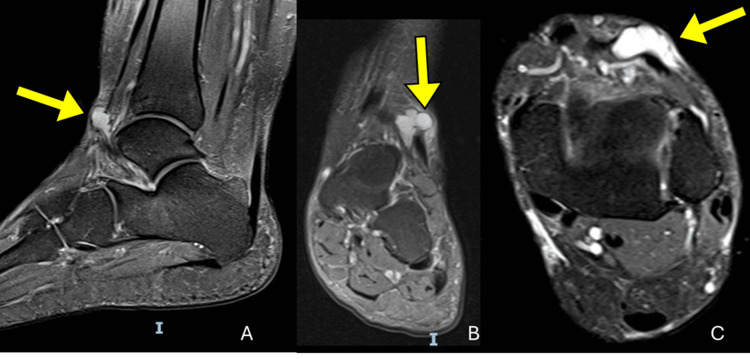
Proton density fat-saturated (PD-FS) MRI of the left ankle demonstrating a ganglion cyst (yellow arrows). (A) Sagittal, (B) coronal, and (C) axial PD-FS images show a well-defined, multilobulated cystic lesion in the anterolateral ankle adjacent to the deep peroneal nerve, superficial to the extensor digitorum longus tendon, also insinuating between the extensor hallucis longus tendon. The lesion exhibits high signal intensity and smooth margins, with no osseous erosion or tendon involvement.
